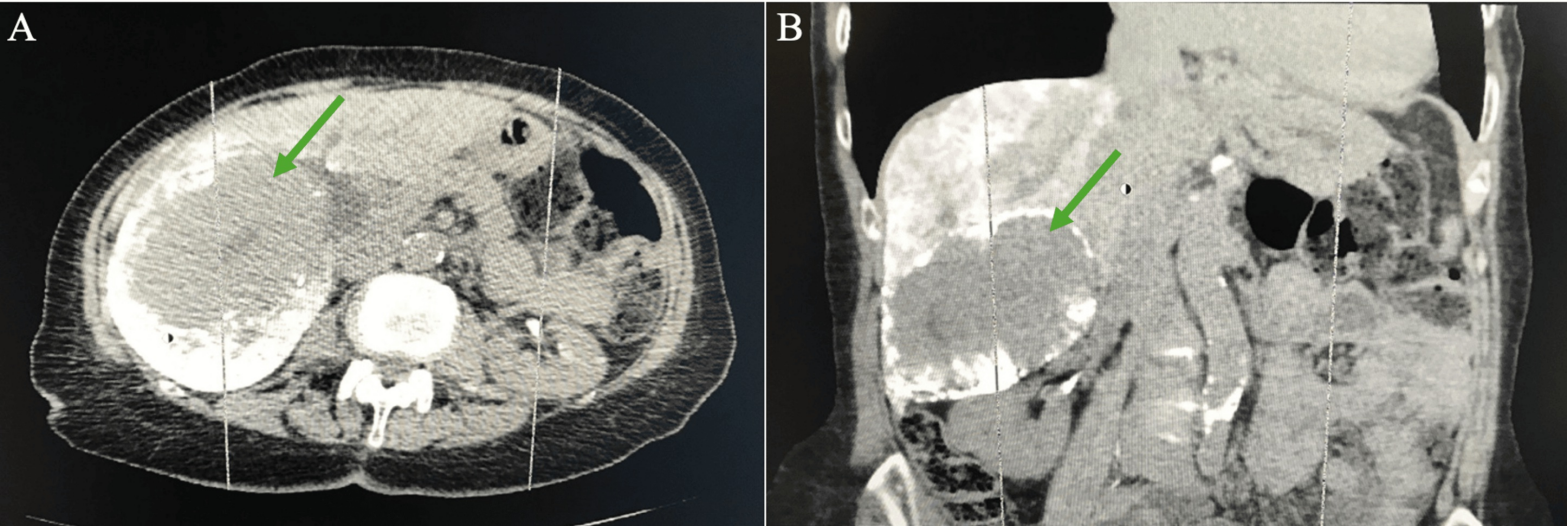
CT of abdomen with transverse (A) and sagittal (B) views taken after liquid embolization with lipiodol and bleomycin, 8 years after initial CT. Hepatic hemangioma (green arrow) measures approximately 10.3 x 7.3 cm.
